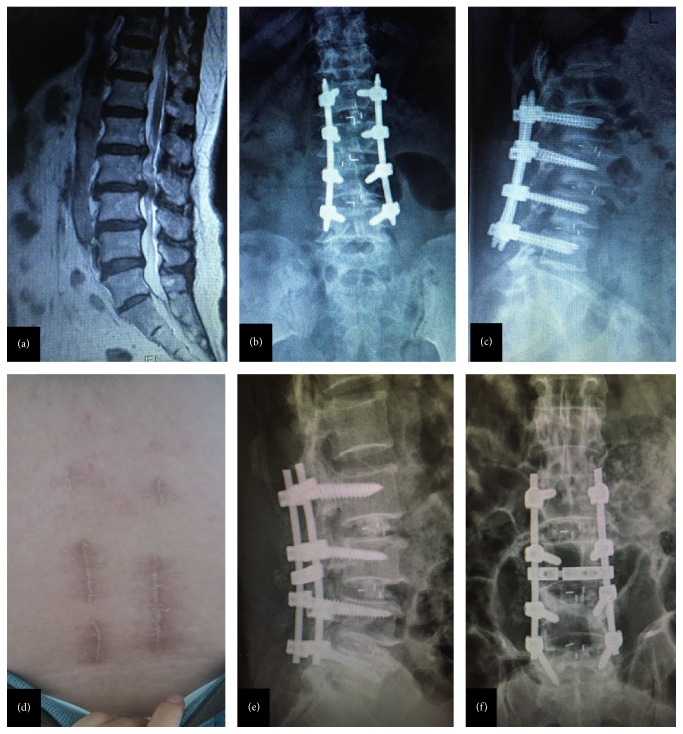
Minimally invasive transforaminal lumbar interbody fusion versus posterior lumbar interbody fusion in three-level spinal stenosis. (a) Preoperative magnetic resonance imaging; (b) anteroposterior fluoroscopy of three-level minimally invasive transforaminal lumbar interbody fusion; (c) lateral fluoroscopy of minimally invasive transforaminal lumbar interbody fusion; (d) minimal incision and skin healing of minimally invasive transforaminal lumbar interbody fusion; (e) lateral fluoroscopy of three-level posterior lumbar interbody fusion; (f) anteroposterior fluoroscopy of three-level posterior lumbar interbody fusion.
